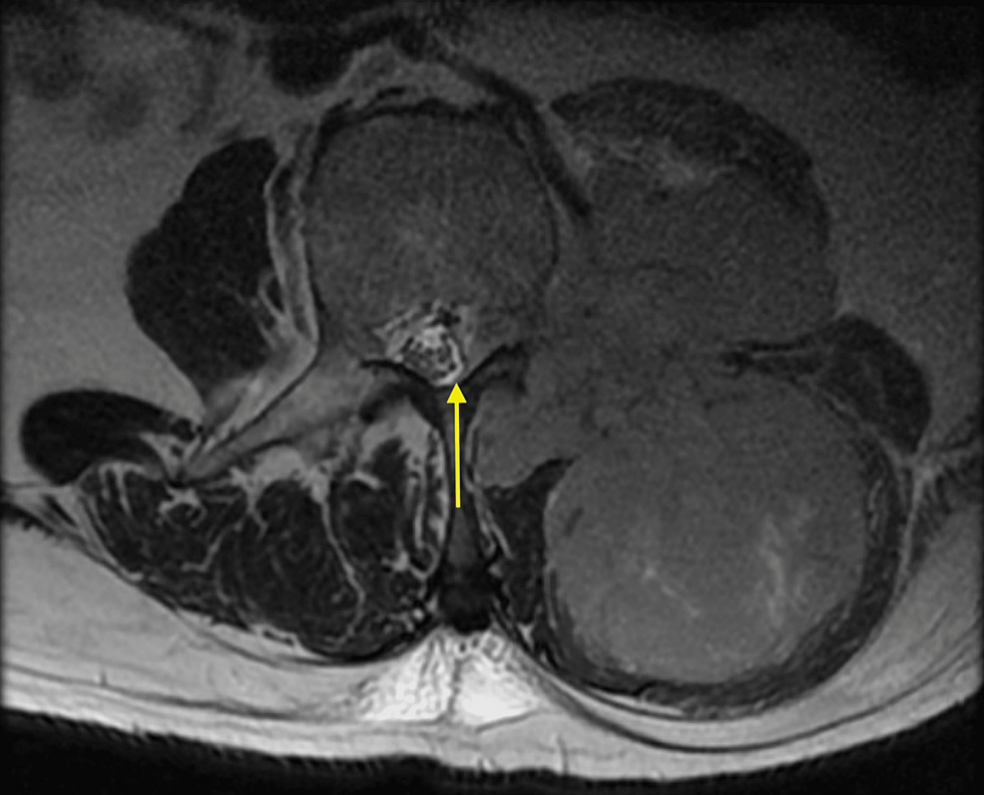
Soft tissue mass protruding into the leftward aspect of the central canal, causing displacement of the thecal sac to the right and resulting in a state of moderate central stenosis shown by the T2-weighted axial view
